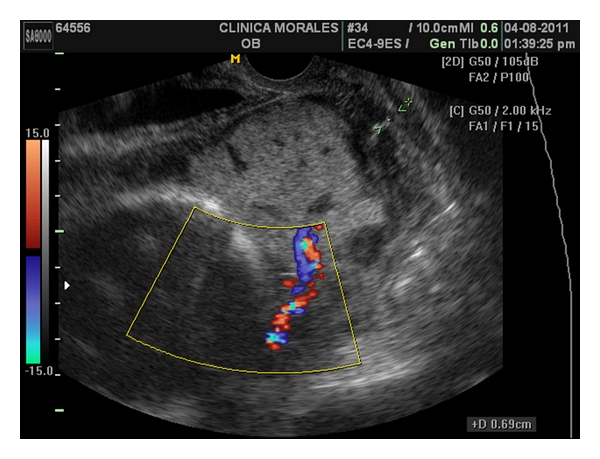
Week 27. Transvaginal colour Doppler ultrasound. The aberrant cotyledon is seen covering the internal os including the umbilical cord insertion. The cervix has become slightly shorter (6,9 mm).
